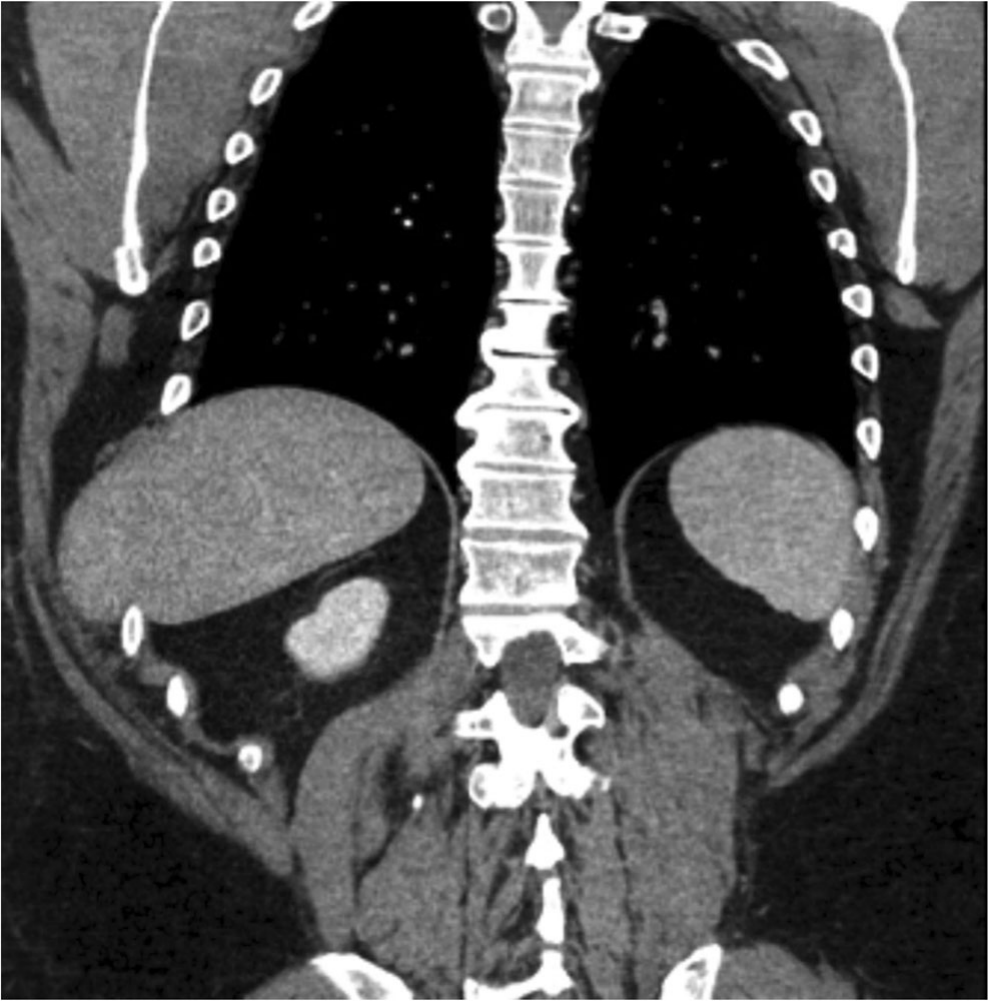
Coronal CT in a patient with a remote history of trauma demonstrates intercostal herniation of the liver
